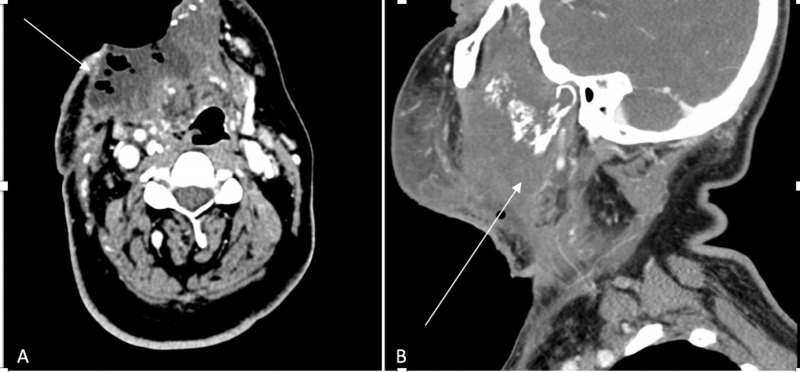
CT scan of neck with contrast after six weeks of therapy CT scan of neck after six weeks of therapy: (A) axial and (B) sagittal views. The white arrow indicates a large necrotic mass involving the patient's right oral cavity, tongue, sublingual space, submandibular space, right masticator space and right pterygopalatine fossa. The mass is more necrotic and has sloughed off.
